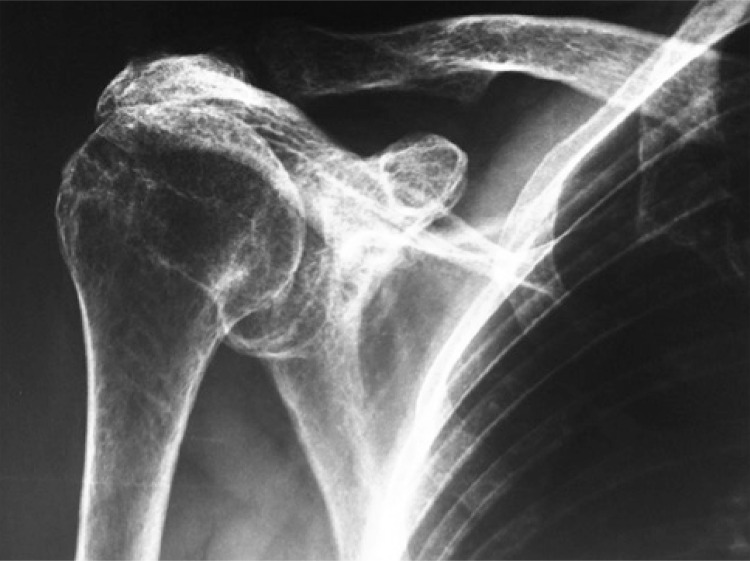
Radiograph of a patient classified as Seebauer IIA
